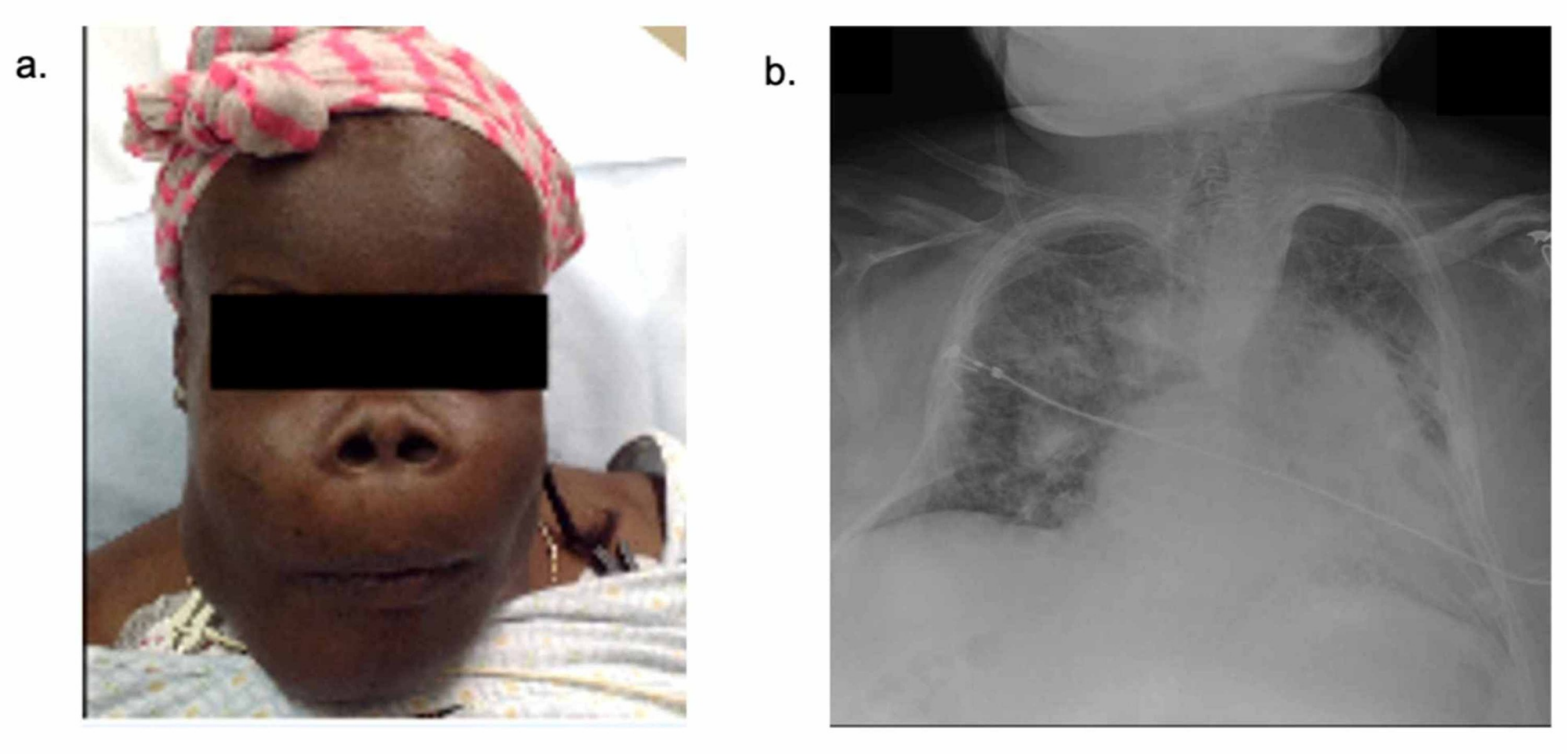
Patient initial presentation on admission Presentation on current admission: a) physical exam notable for severe midface hypertrophy, large forward-facing nostrils, and limited range of motion of the neck; b) chest X-ray on admission demonstrated bilateral restricted lung fields and severe pulmonary edema secondary to hypervolemia
